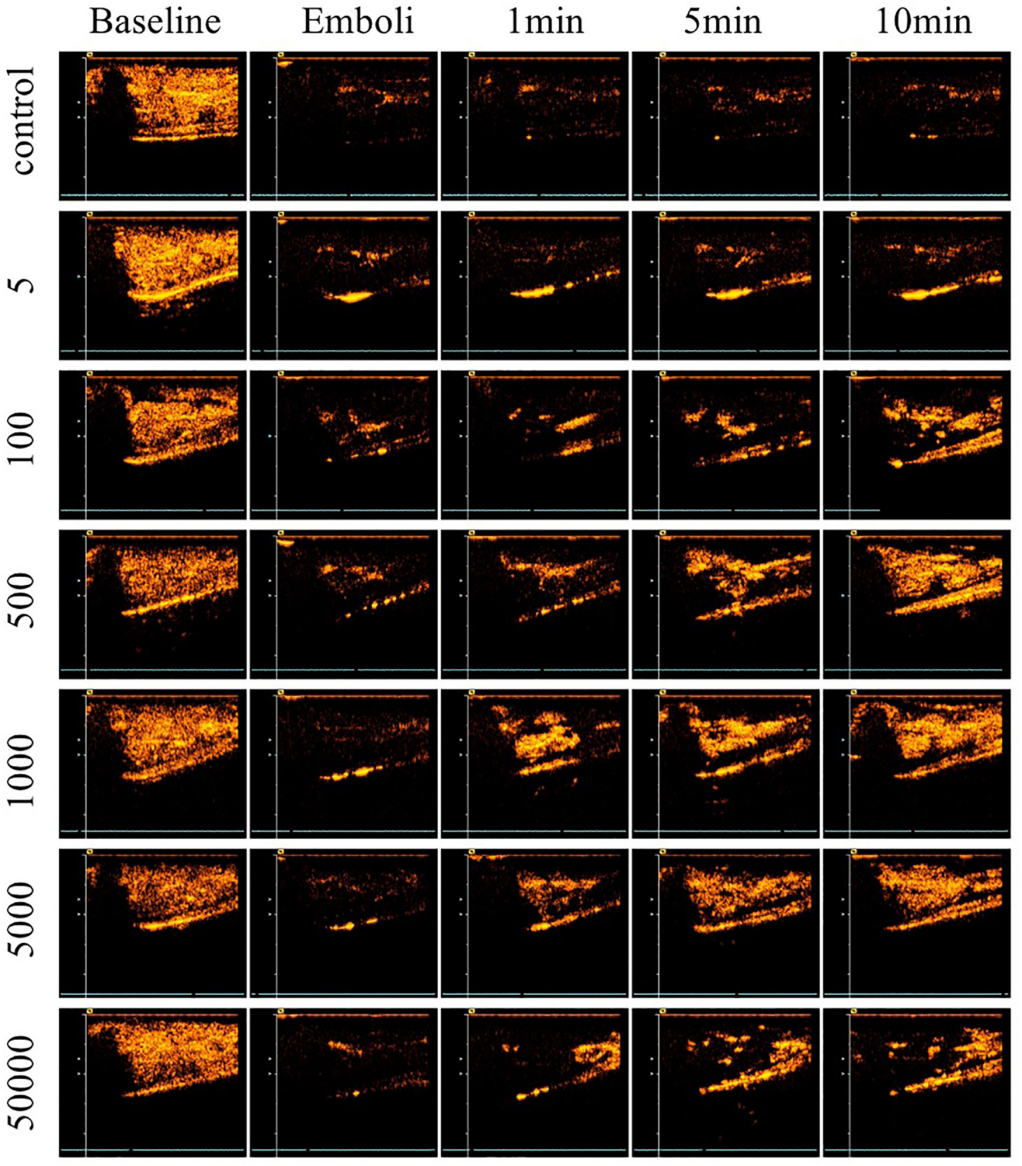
Contrast perfusion images at different experimental stages. Ultrasound pulse length increases from 5 to 50,000 cycles from top to bottom, the control group was given microbubble only with no therapeutic ultrasound. Five experimental stages are listed from left to right: baseline (before treatment), emboli (microvascular embolization), 1, 5, and 10 min post-therapeutic ultrasound.
